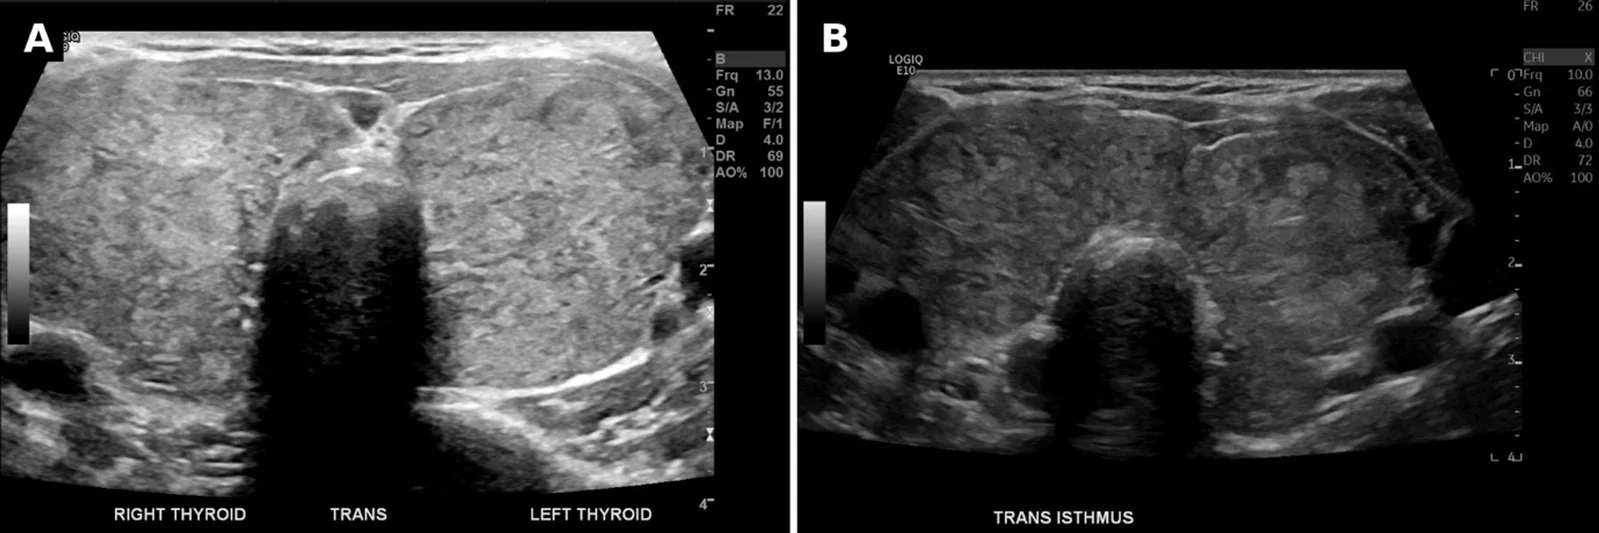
Serial thyroid imaging demonstrating progressive gland enlargement over time (A) Transverse ultrasound, Year 1 (12/2018): diffusely enlarged, heterogeneous thyroid gland without evidence of a discrete nodule (right lobe 5.2 × 2.7 × 2.4 cm; left lobe 4.9 × 2.7 × 2.4 cm). (B) Transverse ultrasound, Year 3 (10/2020): markedly heterogeneous and hypervascular gland compatible with thyroiditis, with a new 1.4 × 1.1 × 1.1 cm hyperechoic right lobe nodule (right lobe 7.0 × 3.0 × 2.7 cm; left lobe 7.1 × 4.3 × 2.6 cm).
